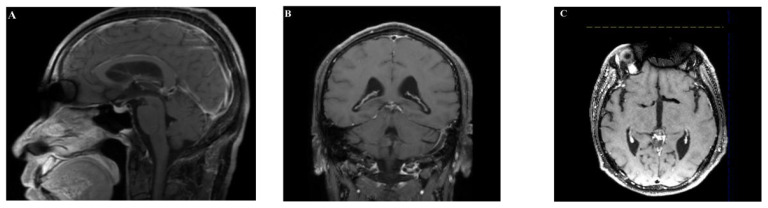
(A–C) Postoperative follow-up magnetic resonance imaging.
